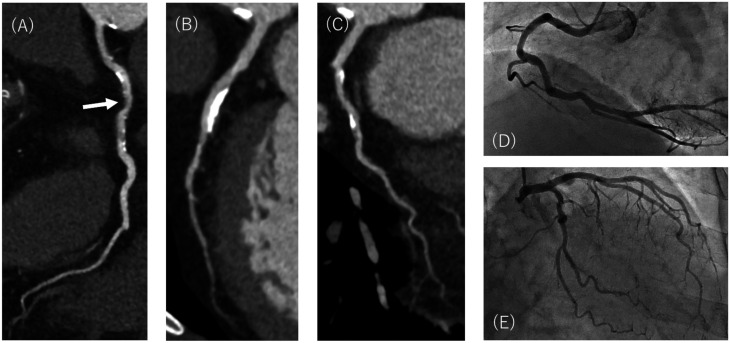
CT angiography images of (A) right coronary, (B) left anterior descending, and (C) left circumflex arteries in a 75-year-old female with atypical chest pain reconstructed with model-based iterative reconstruction. Middle segment of the right coronary artery was diagnosed as 50% to 69% stenosis partly from image noise (arrow). (D, E) Catheter-based conventional coronary angiography demonstrates no significant stenosis in all arteries.
